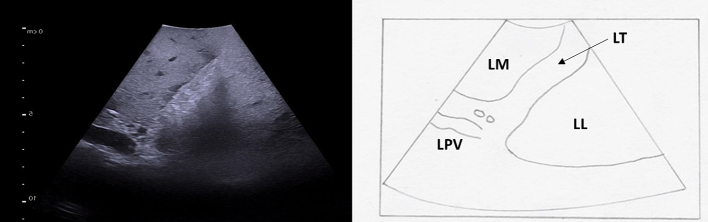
The ligamentum teres (round ligament) is seen as a hyperechoic zone at the free edge of the falciform ligament on intraoperative ultrasound. The left portal vein terminates here, giving off its branches to segment 3 and 4b. LT ligamentum teres, LM left medial, LL left lateral, LPV left portal vein.
